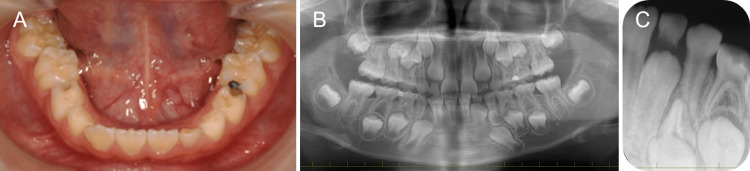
Initial examination at the age of seven years and four months (A) Intraoral photograph; (B) Panoramic radiograph; (C) Periapical radiograph
